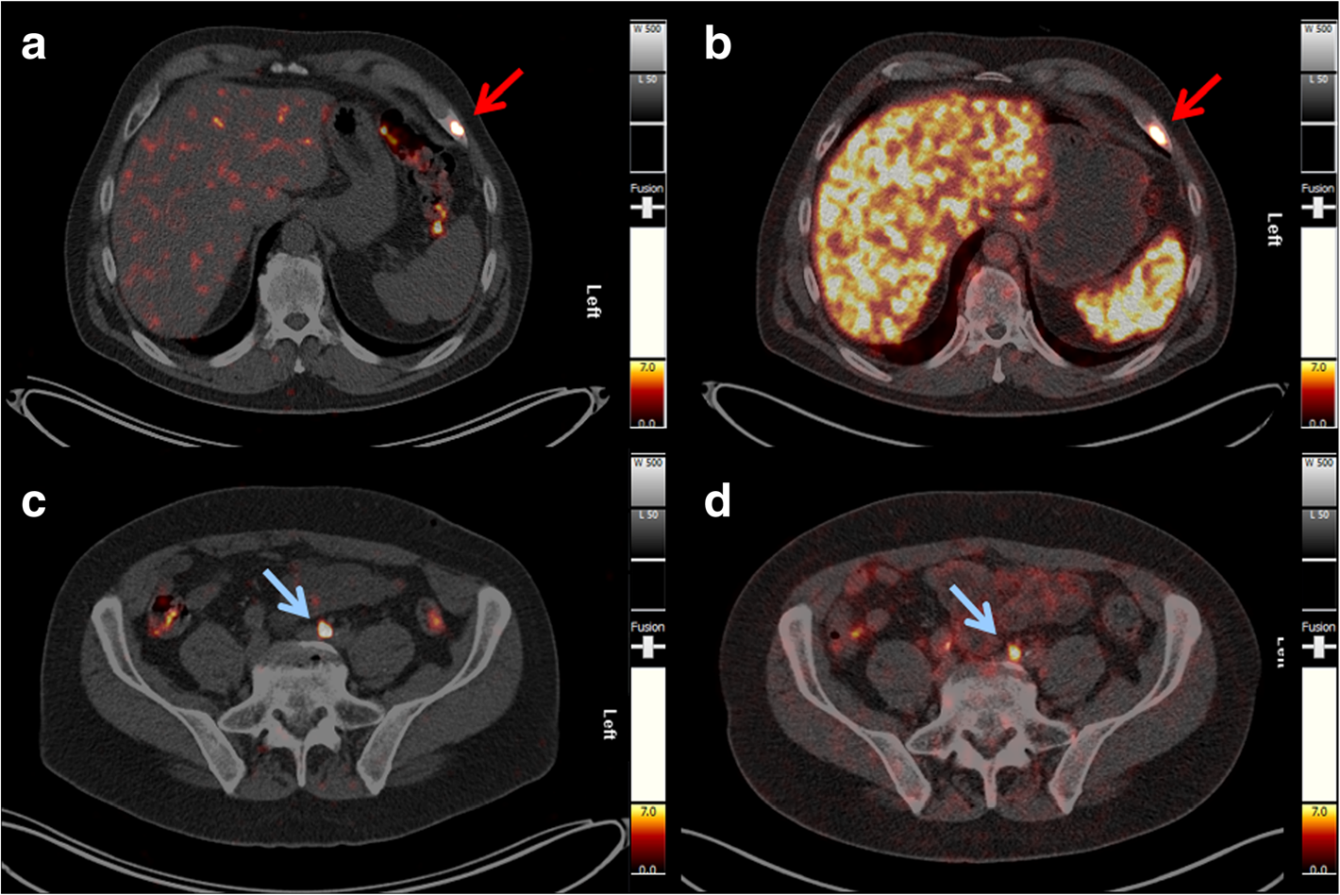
Comparison of PET/CT scans obtained with ^152^Tb-PSMA-617 and ^68^Ga-PSMA-11, respectively. a, c PET/CT image acquired 18.5 h after injection of 140 MBq of ^152^Tb-PSMA-617. b, d PET/CT image acquired 45 min after injection of 160 MBq of ^68^Ga-PSMA-11. Red arrows show a bone metastasis in the ventrolateral part of the left 7th rib, and blue arrows show a small lymph node metastasis along the left common iliac artery
